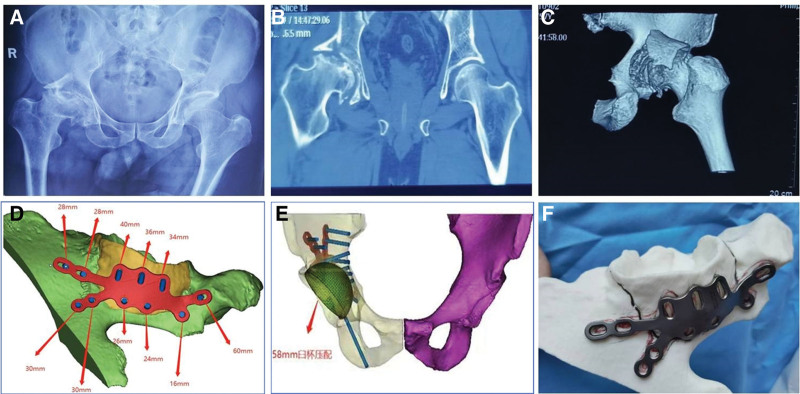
Preoperative X-ray, CT, and 3D printing design. (A–C) A fresh fracture of the posterior wall of the right acetabulum and femoral head necrosis. (D and E) Three-dimensional modeling in MIMICS software, designing personalized bone plate and evaluating the size of the acetabular cup. (F) 3D printing the acetabulum model and personalized bone plate.
